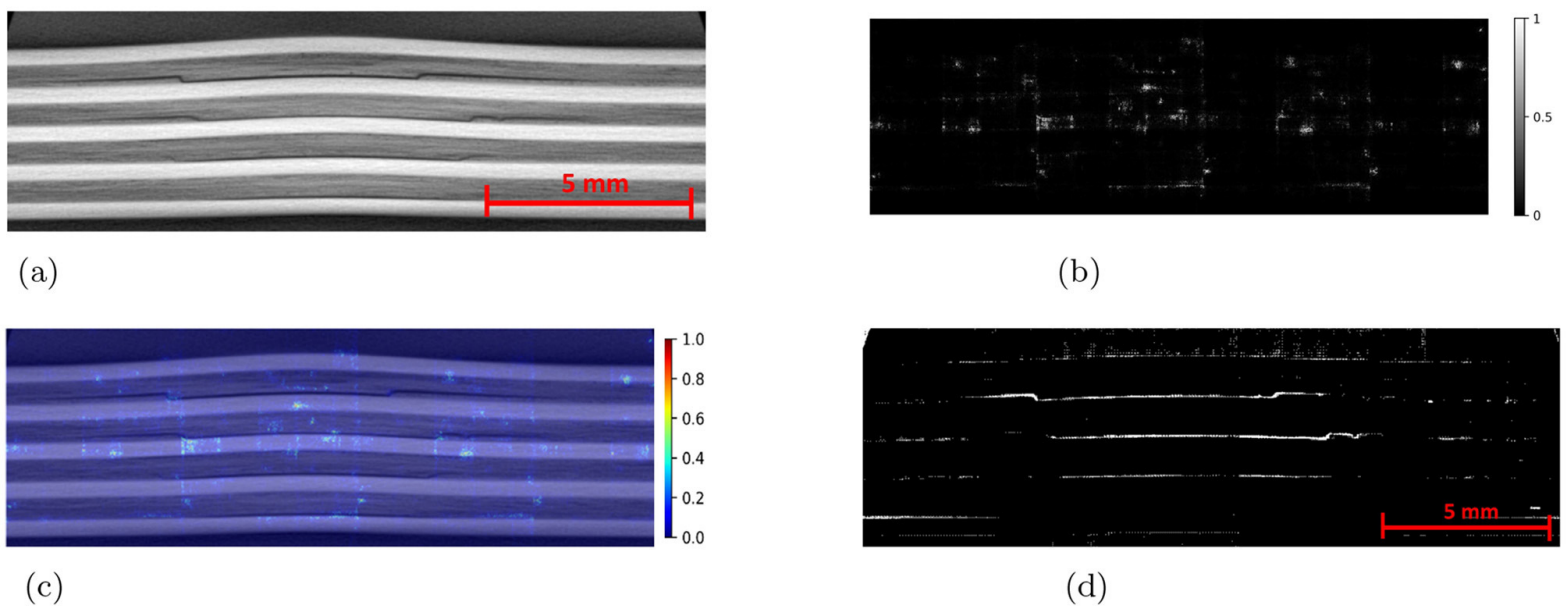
An example CT slice of the damaged plate with 7.5 Joules impact energy is shown in (a). The attribution map is produced by applying the IG method to the trained SAM. The overlap of the attribution map and CT slice in (c) suggests that the SAM has very low interpretability. (a) CT slice with impact energy of 7.5 Joules. (b) Attribution map. (c) Overlap of attribution map and CT slice. (d) Predicted mask.
